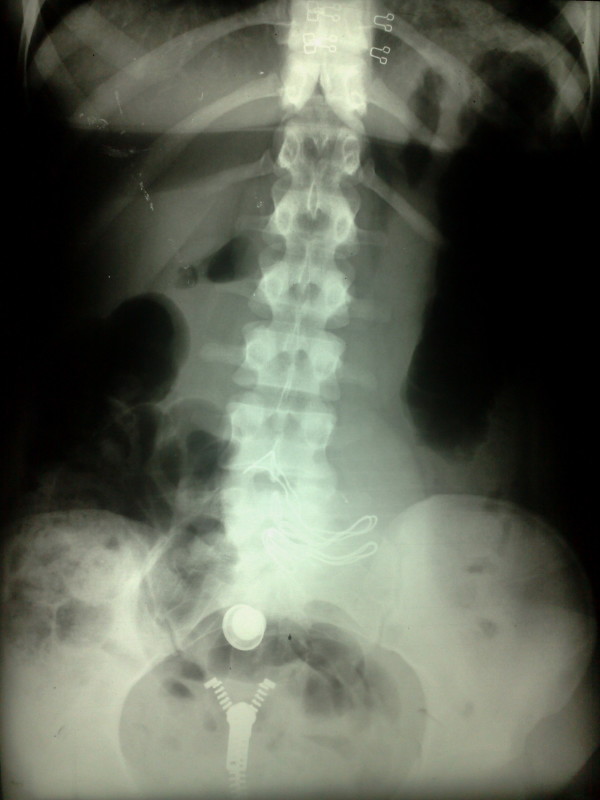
Plain X-ray of the abdomen showing the radio-opaque marker of the retained gauze in the center of abdomen.
